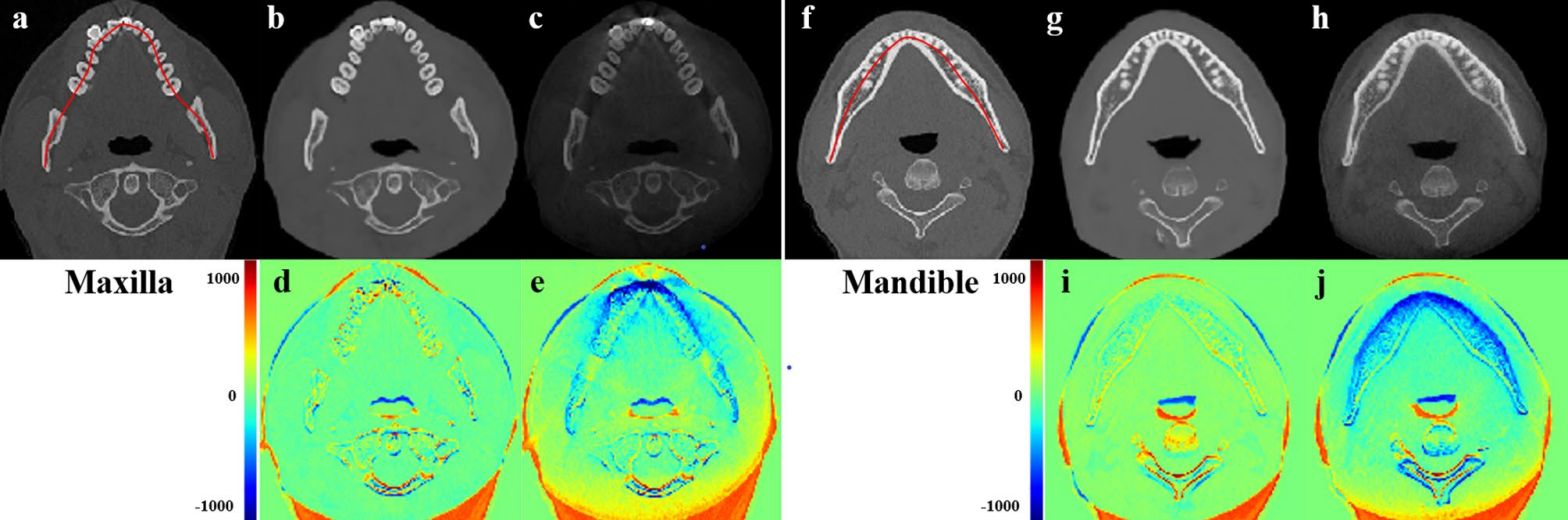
Axial slices of BMD images from the original QCT (a,f), QCBCT (b,g), and CAL_CBCT (c,h) images and their subtractions from the original QCT images at the maxilla (d,e) and mandible (i,j). The red curves shown in the QCT images are the dental arch used to obtain the BMD profiles.
